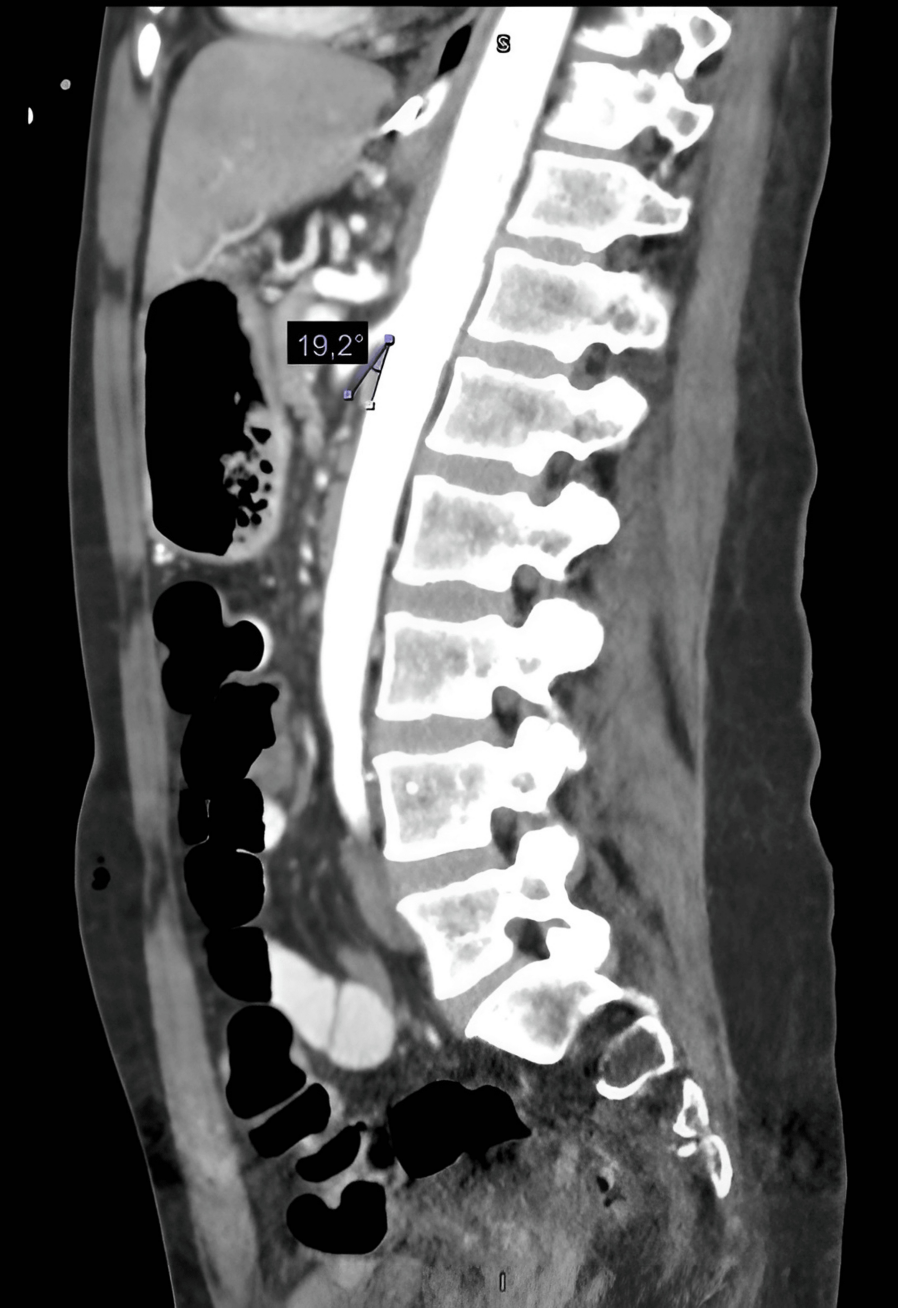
Sagittal CT scan. Measurement of the aortomesenteric arch (aortomesenteric angle measures 19.2°). CT: computed tomography
